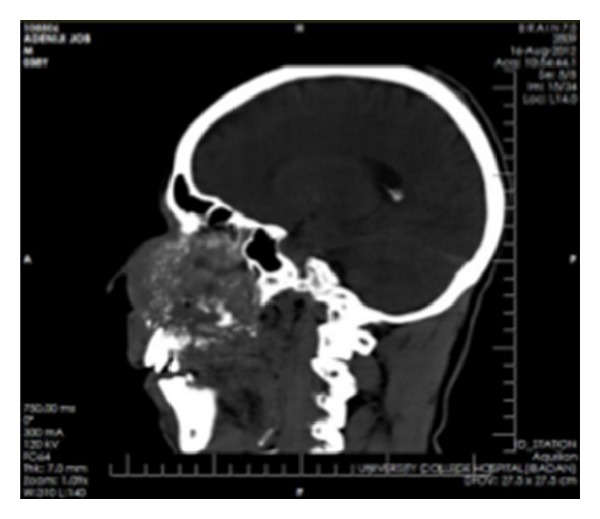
Sagittal computerized tomography (CT) showing destruction of the maxilla and nasal tissues with “floating” upper teeth.
